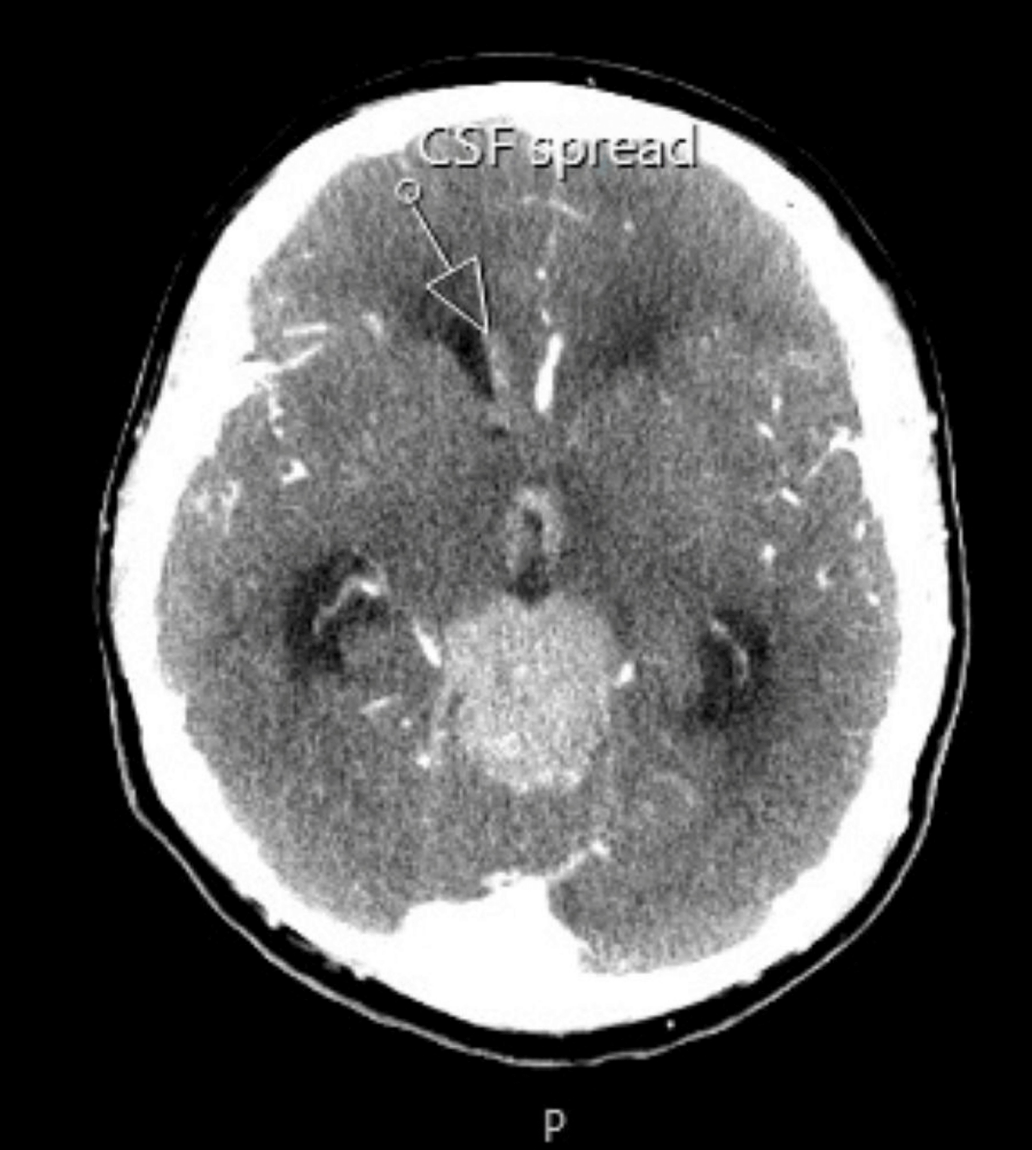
CT of the pituitary with contrast. White arrow: cerebrospinal fluid spread.
